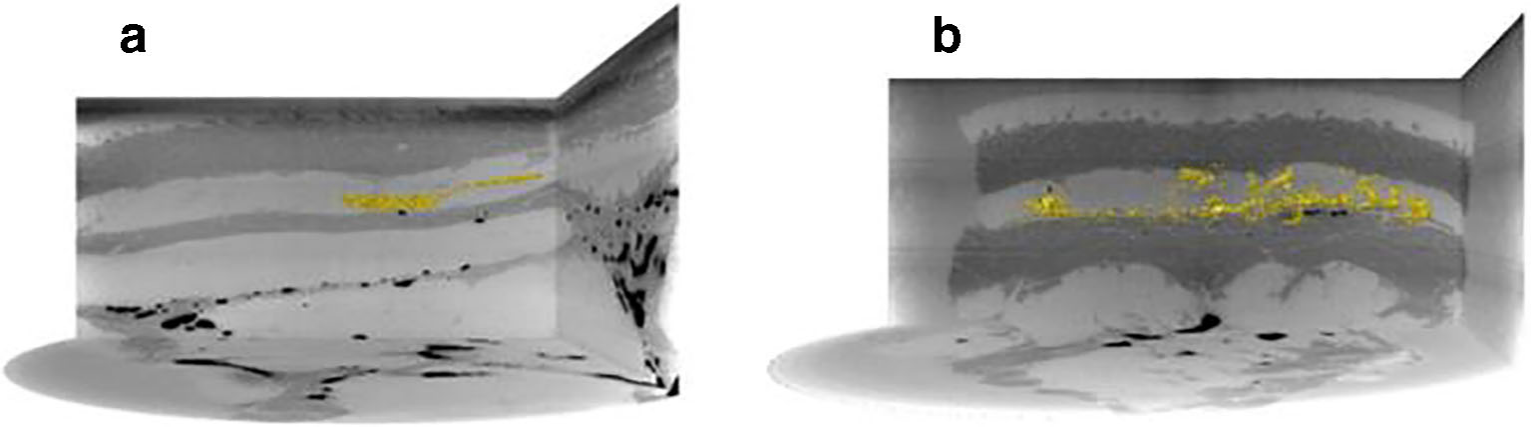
Volume renderings of PLG microspheres in the absence of contrast agent (yellow) are overlaid onto orthogonal reconstructed CT sections of the tissue sample, for (A) aqueous and (B) non-aqueous vehicles for images acquired using the LFV setup. The cross-sectional diameter represented here is 85 mm.
